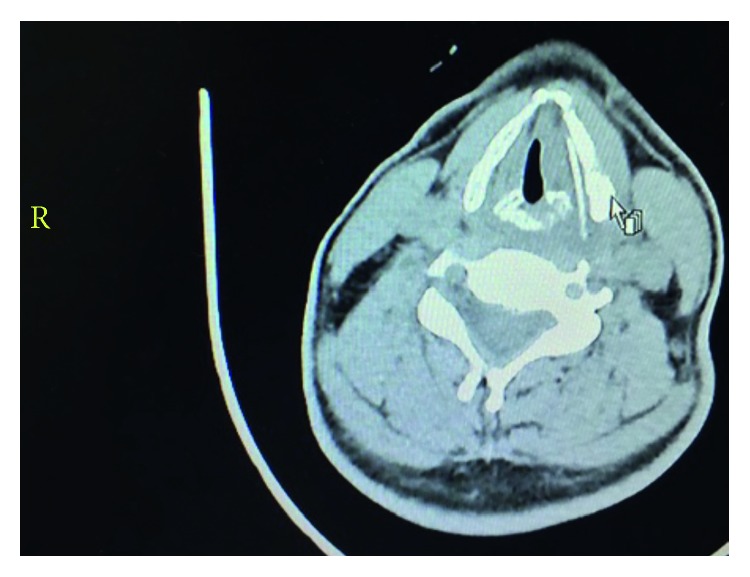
CT scan of the patient's neck.
